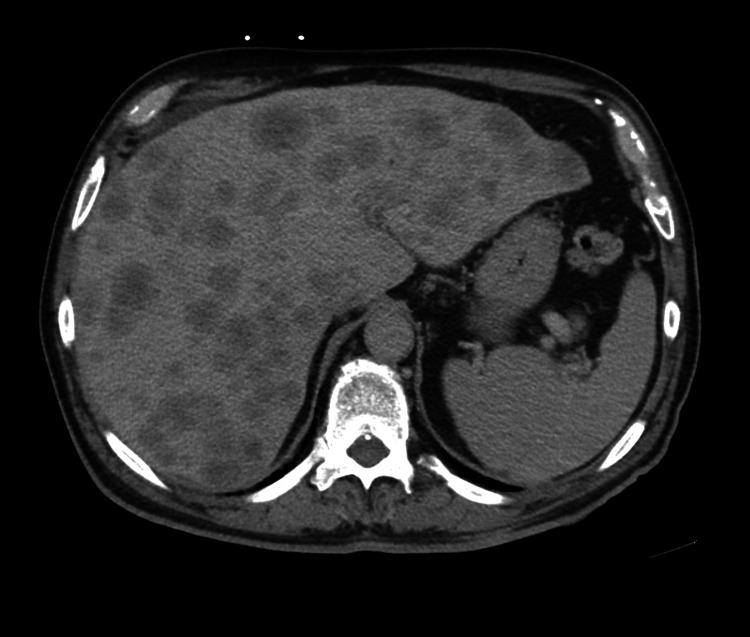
Contrast-enhanced CT of the abdomen shows innumerable metastatic lesions in both lobes of the liver.
